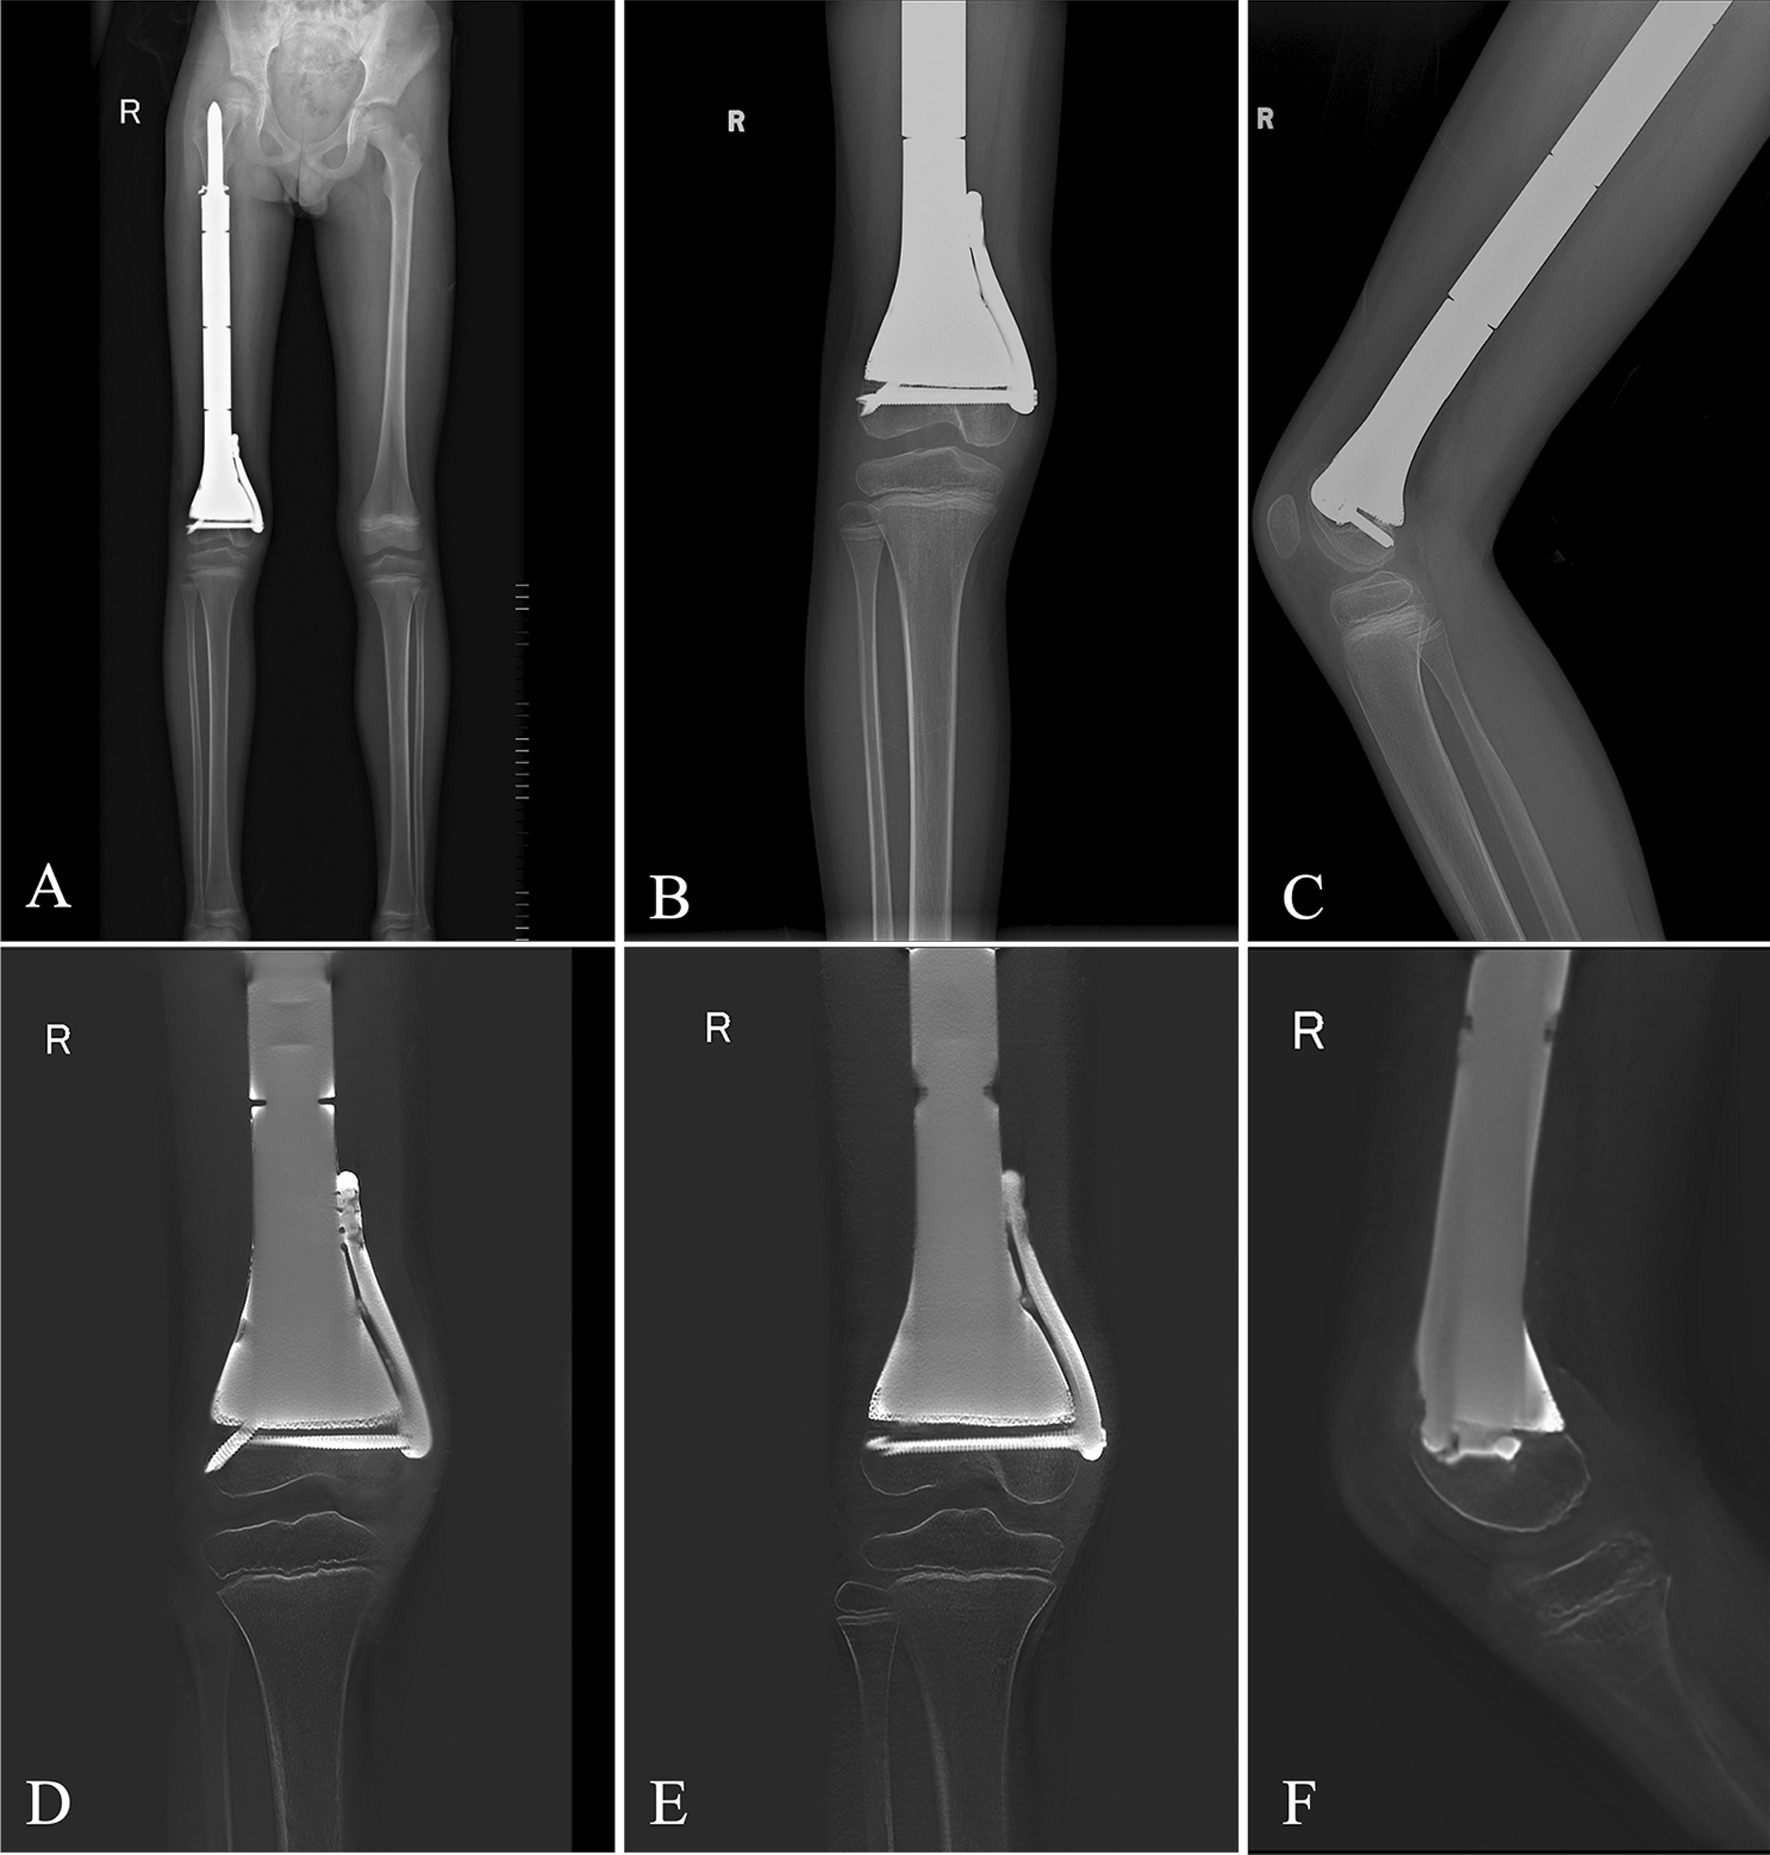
Postoperative radiographs. A–C plain radiograph shows the proper position of the 3D-printed endoprosthesis; D–F digital tomosynthesis image shows good osseointegration at the interface between the host bone and the porous surface
